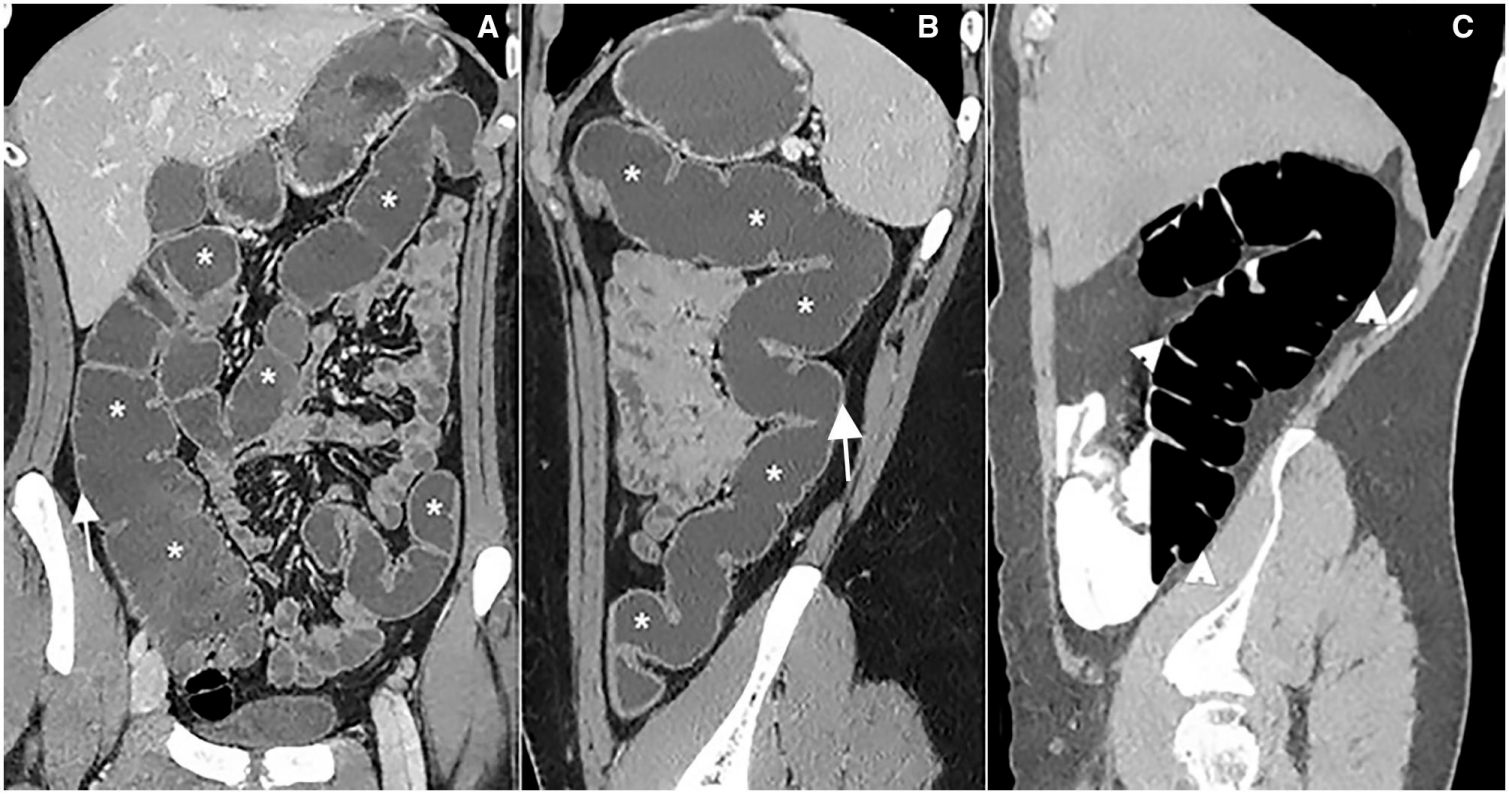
CT with water enema (CT-WE) shows good distension of the large bowel (asterisks) in coronal (A) and sagittal (B) images showing an excellent contrast between the hypodense water-filled lumen and the colonic wall (white arrows in A and B). (C) This technique allows high quality visualization of the entire colonic wall, better than that obtained with CT-colonography, as shown in C (arrowheads).
